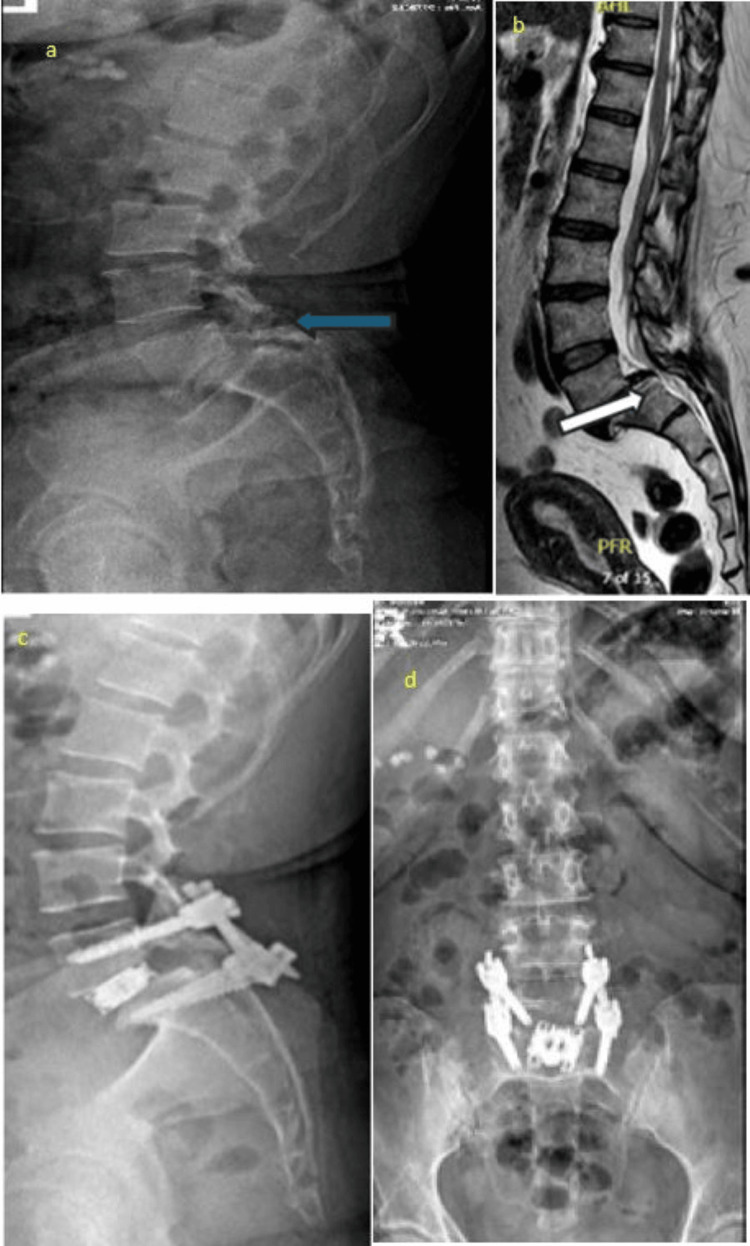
Grade 2 spondylolisthesis a) A 56-year-old female presented with low back pain and left lower-extremity symptoms. Unilateral biportal endoscopic transforaminal lumbar interbody fusion (UBE-TLIF), with unilateral laminotomy and bilateral decompression, was performed using a dual-direction expandable titanium cage via a left-sided approach. Preoperative lateral X-ray shows lower lumbar degenerative changes and grade 2 L5-S1 spondylolisthesis, with collapse of the intervertebral disc space. b) Preoperative sagittal T2-weighted magnetic resonance imaging (MRI) of the lumbosacral spine showing grade 2 spondylolisthesis and associated disc degeneration at the L5-S1 level (white arrow). c) Postoperative lateral X-ray showing the dual-direction expandable cage inserted at the L5-S1 disc space. The intervertebral space is expanded following cage insertion, demonstrating excellent reduction, improved alignment, and restoration of both disc height and foraminal height. Pedicle screws are in place. d) Postoperative anteroposterior (AP) X-ray showing the dual-direction expandable cage in situ, with a large footprint providing stable interbody support. Pedicle screws are symmetrically placed, confirming satisfactory alignment and fixation.
